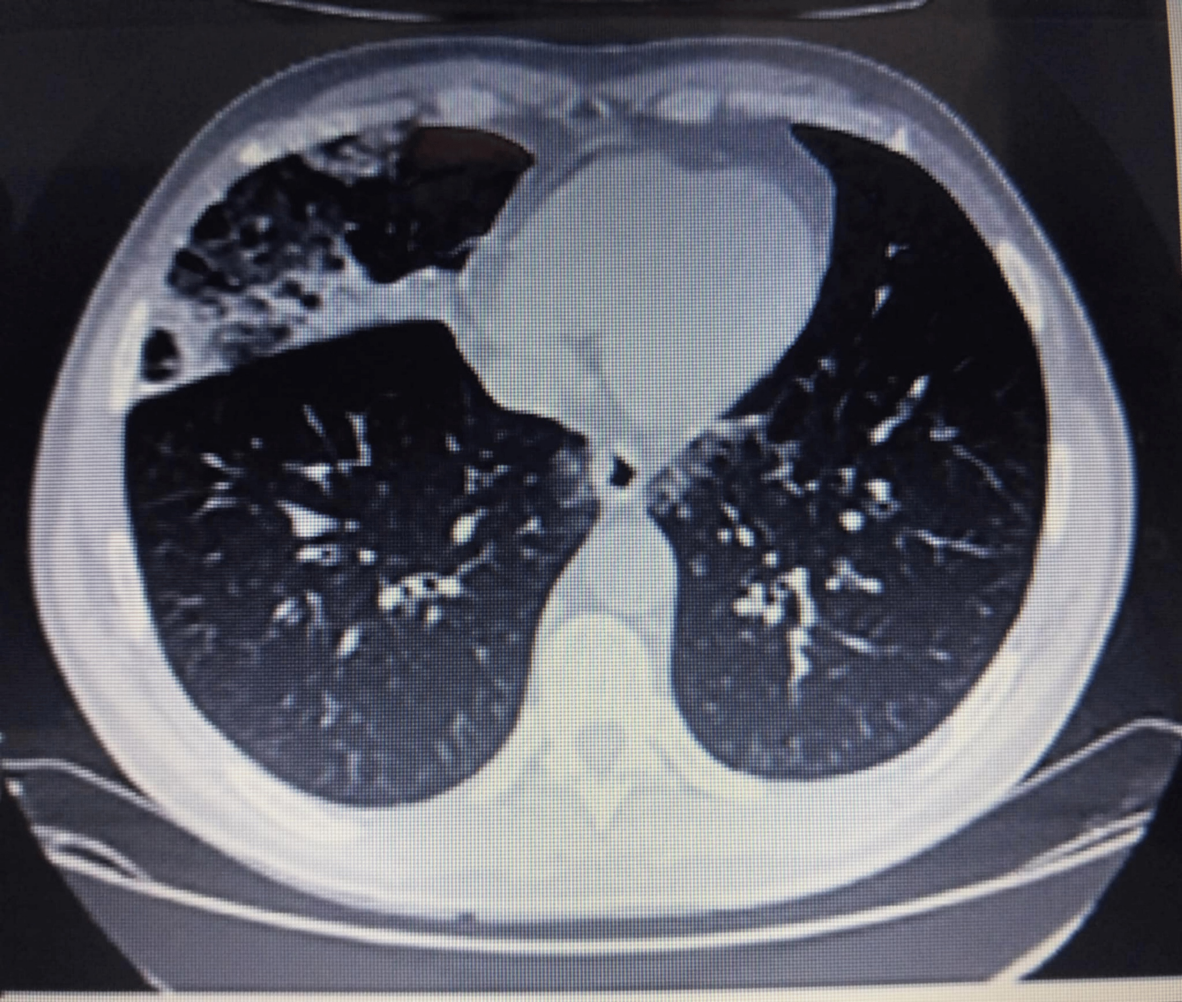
Thoracic CT scan showing bilateral pleural effusion with right-sided predominance, parenchymal consolidations, and ground-glass opacities suggestive of infection CT: computed tomography
